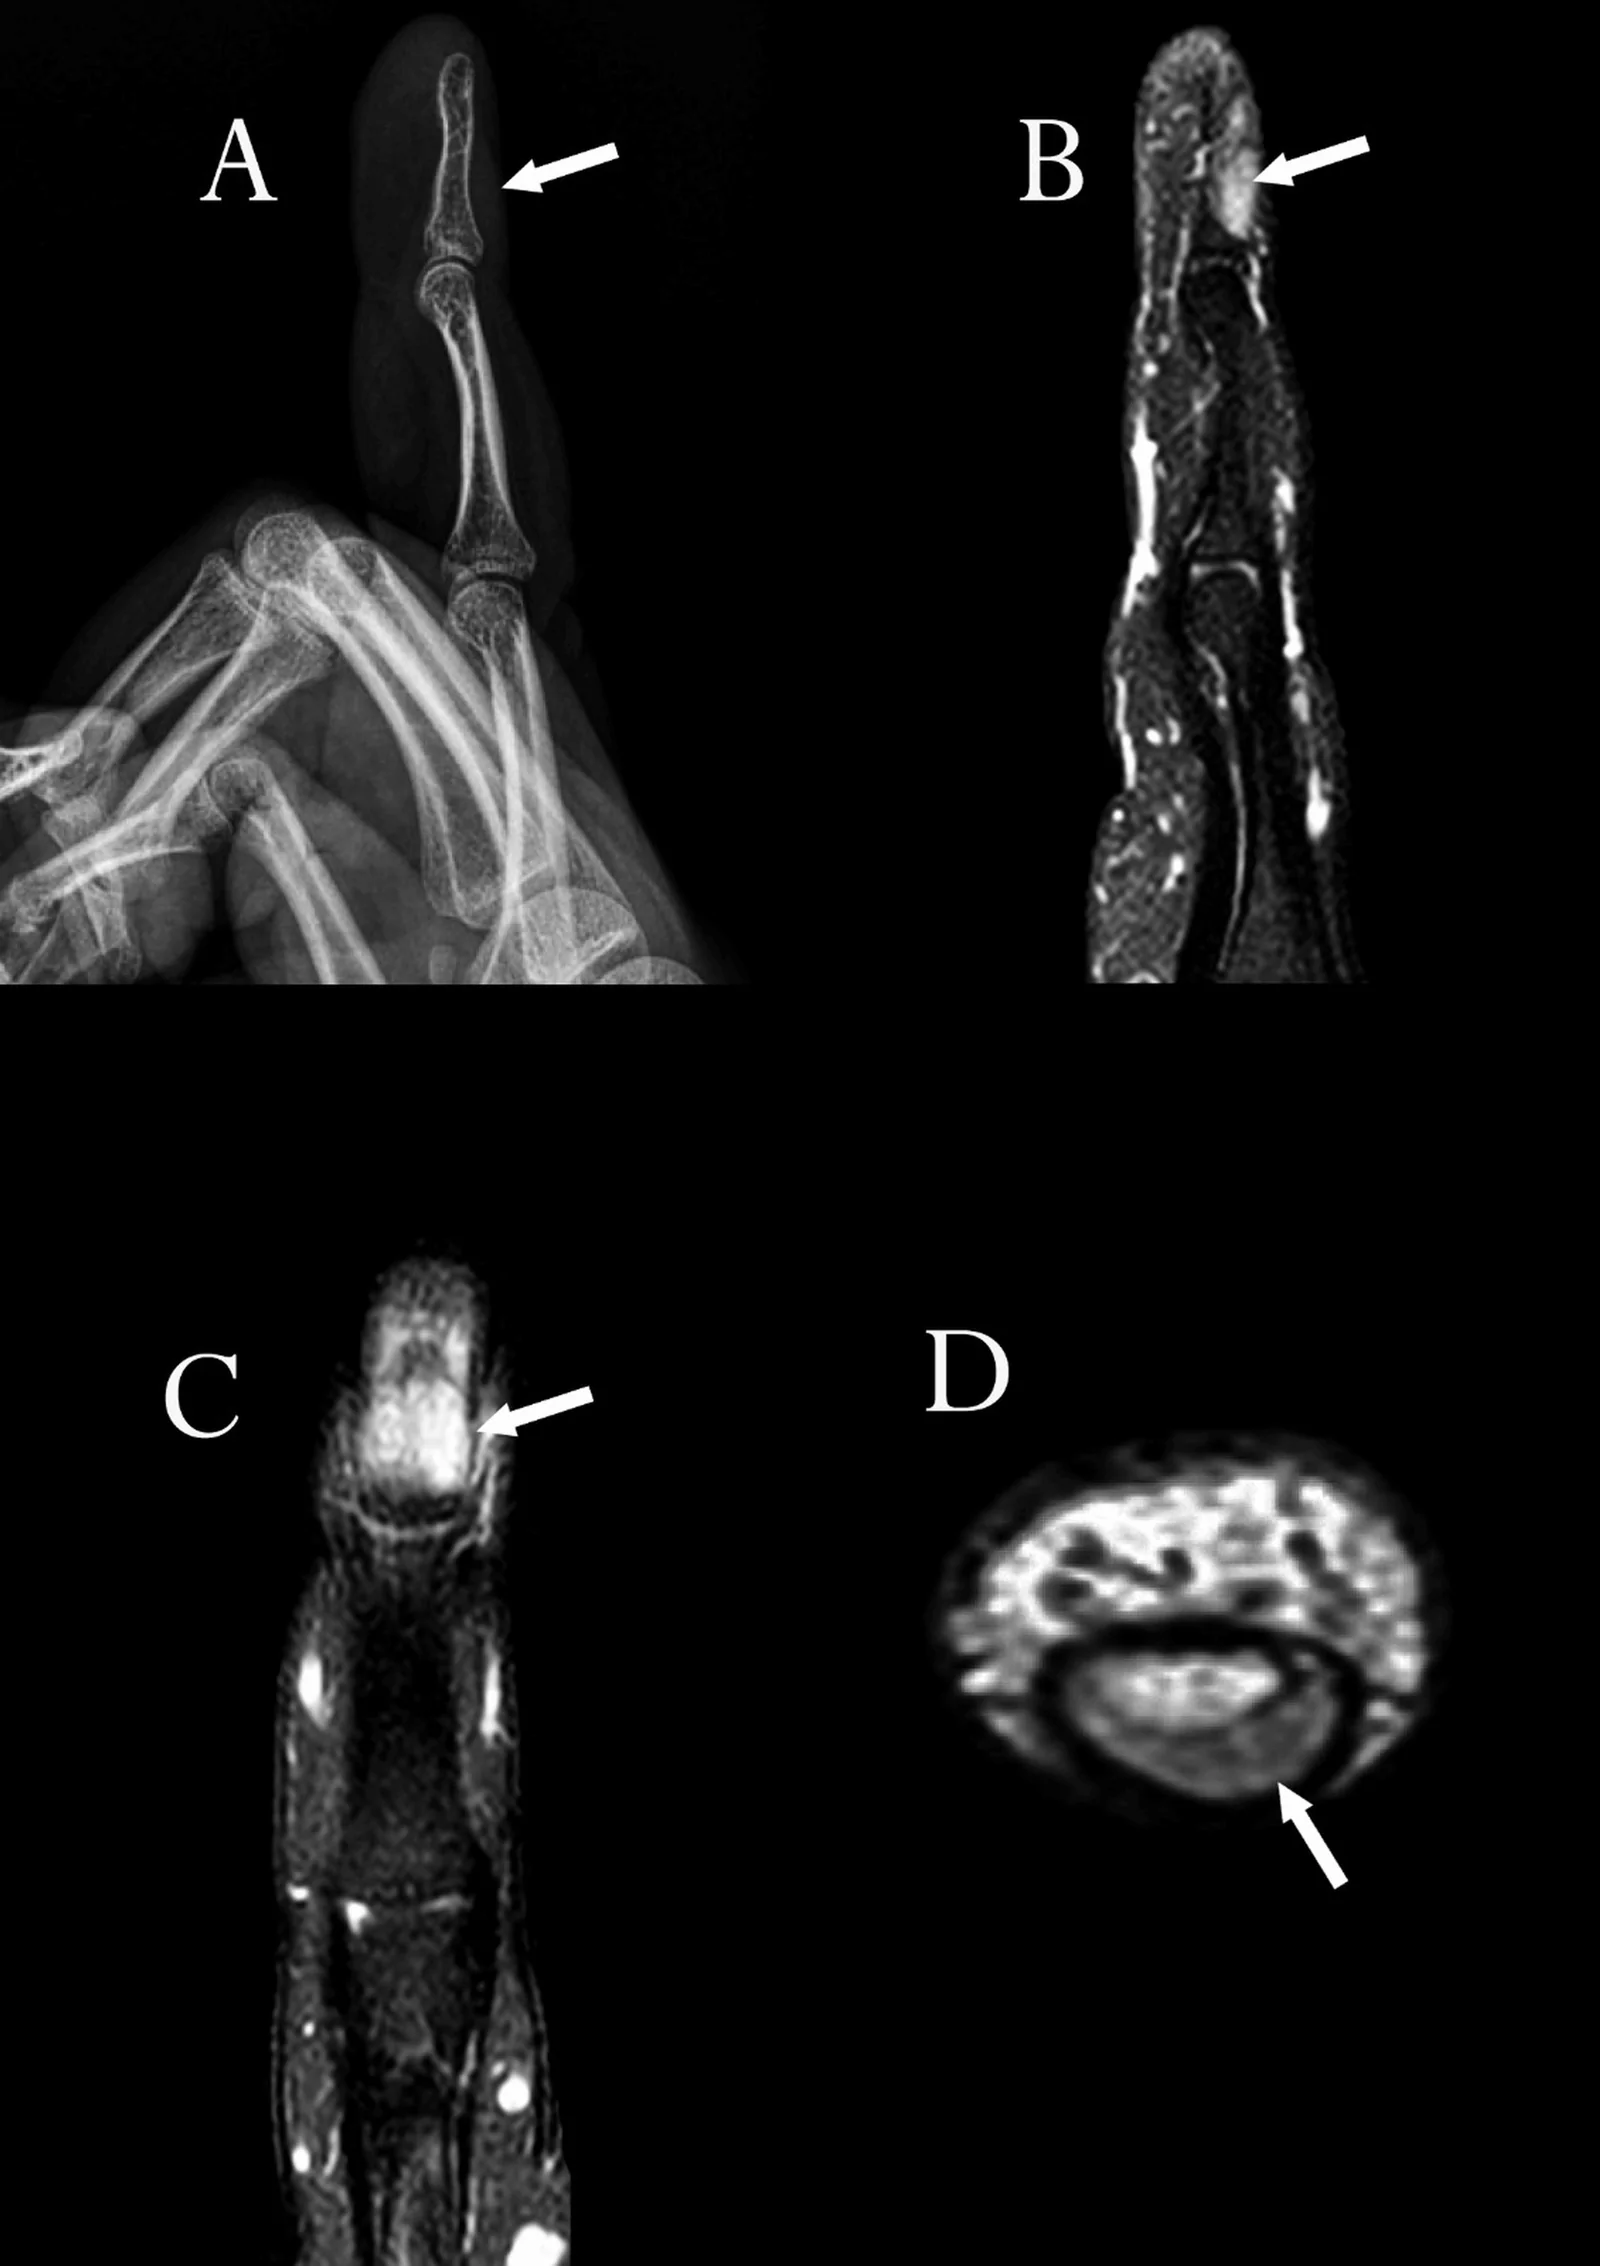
(A) Plain radiograph of the right hand demonstrating no bony erosion, periosteal reaction, or calcification at the distal phalanx of the ring finger, effectively excluding bony pathology. (B) Sagittal T2-weighted MRI demonstrating a well-defined hyperintense subungual lesion at the distal phalanx of the ring finger. (C) Coronal T2-weighted MRI showing the hyperintense subungual lesion. (D) Axial MRI cross-section confirming the subungual location of the lesion and delineating its relationship to adjacent structures. No cortical erosion is identified on any sequence.
